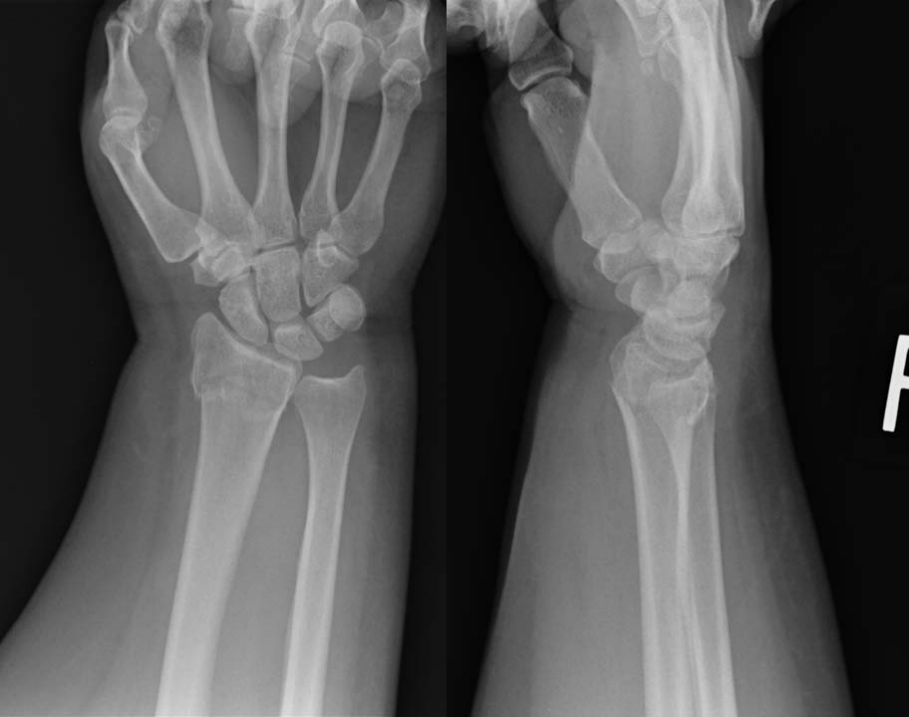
Initial radiographic view of the right wrist following failure of reduction in 1 week.
